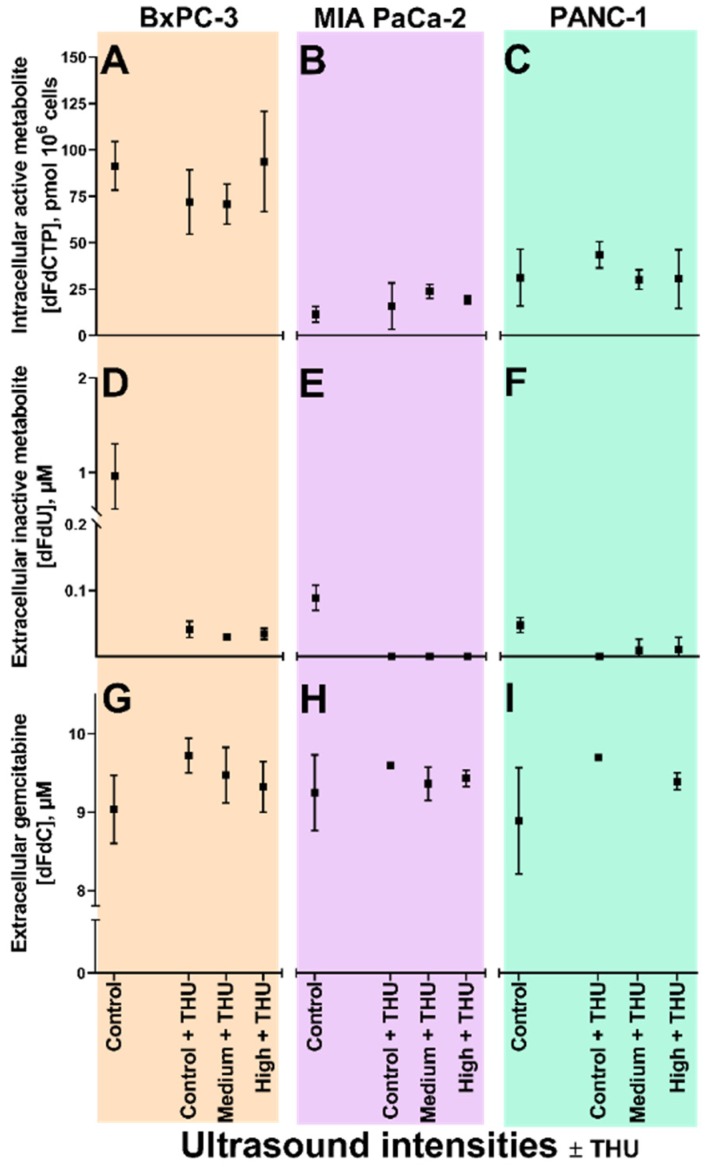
Gemcitabine uptake and metabolism following sonoporation of PDAC cell lines with inhibited cytidine deaminase. Extracellular gemcitabine (dFdC, panels G, H, I), extracellular inactive gemcitabine metabolite (dFdU, panels D, E, F), and intracellular active gemcitabine metabolite (dFdCTP, panels A, B, C), in BxPC-3 (orange), MIA PaCa-2 (purple) and PANC-1 (green) cell lines following 60 min co-incubation with 10 µM gemcitabine +200 µM tetrahydrouridine (THU), 3.84 × 10^6^ ppmL Sonazoid^®^ microbubbles and 5 min ultrasound (US at two acoustic intensities (Medium^1^, High^2^) and no US (Control) in Petakas. 60 min incubation with 10 µM gemcitabine without US included as control (leftmost data point in all panels). Results displayed as mean ± SD (*n* = 3–4). No significant differences between means of Control + THU vs. Medium + THU vs. High + THU (Unpaired students *t*-tests). ^1^ Medium US intensity: 2.0 MHz, MI 0.2, 80 cycles, DC 1.8%, *I*_SPPA_ 3 W/cm^2^ and *I*_SPTA_ 50 mW/cm^2^; ^2^ High US intensity: 2.0 MHz, MI 0.378, 160 cycles, DC 3.6%, *I*_SPPA_ 10 W/cm^2^ and *I*_SPTA_ 358 mW/cm^2^.
